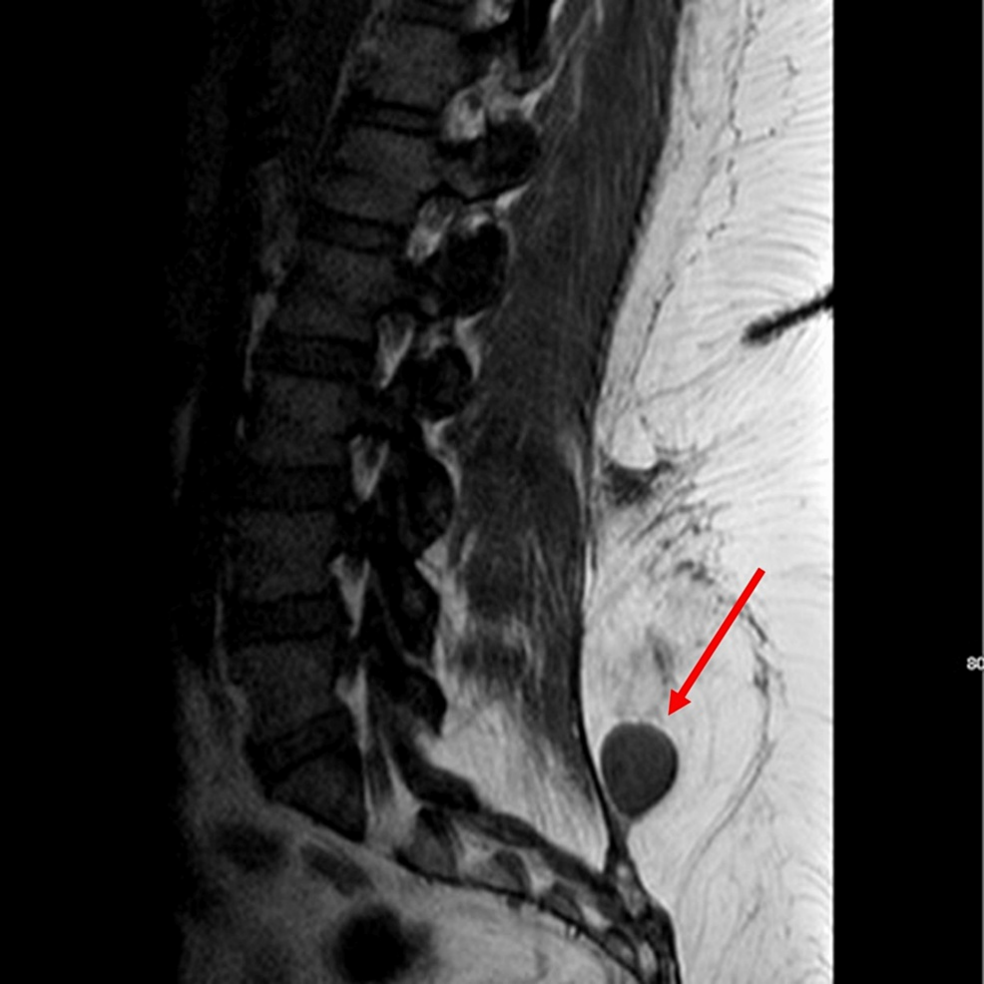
MRI lumbar spine with and without contrast Solid soft tissue nodule in the subcutaneous fat of the lower back at the level of the S1 segment (arrow). The lesion is superficial to the posterior lumbar fascia but demonstrates solid enhancement and is compatible with a neoplasm.
